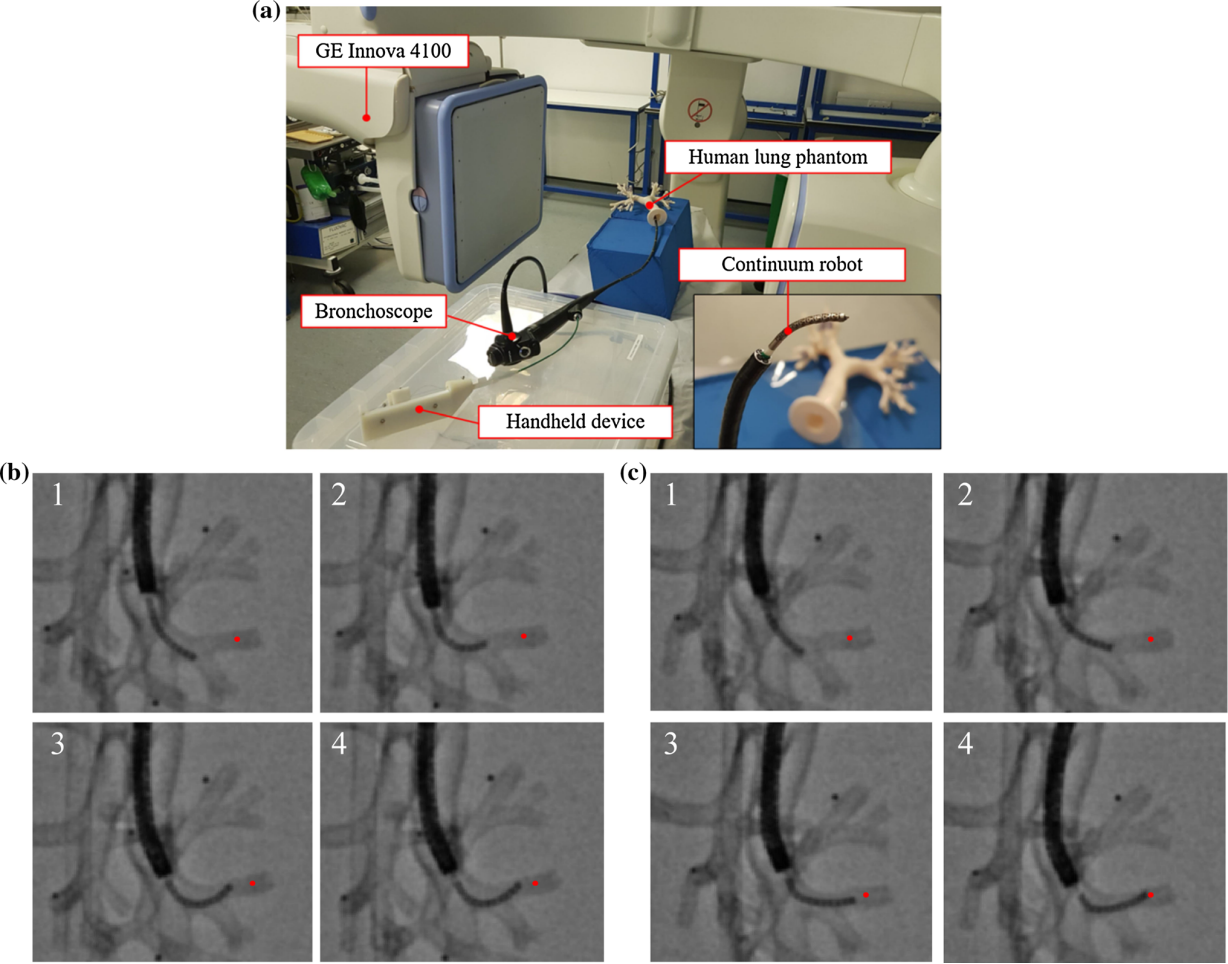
Optimization validation. a Experimental validation setup. b X-ray images of the intervention performed with the constant-curvature CR. c X-ray images of the intervention performed with the optimized CR. The desired target is marked in red
